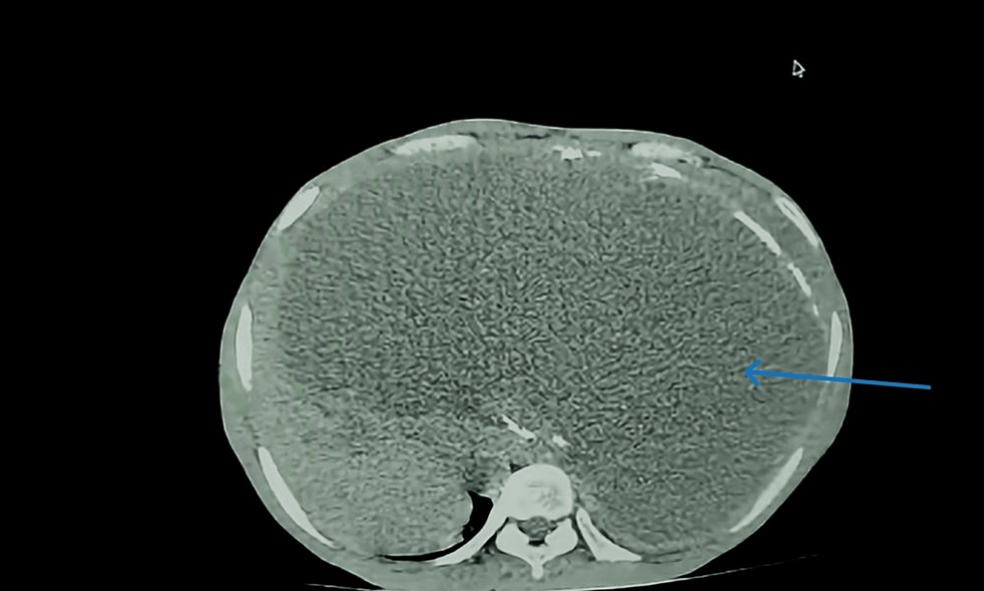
A CT scan of the abdomen showing a large fluid-filled lesion occupying most of the abdomen The blue arrow represents the hydatid cyst
